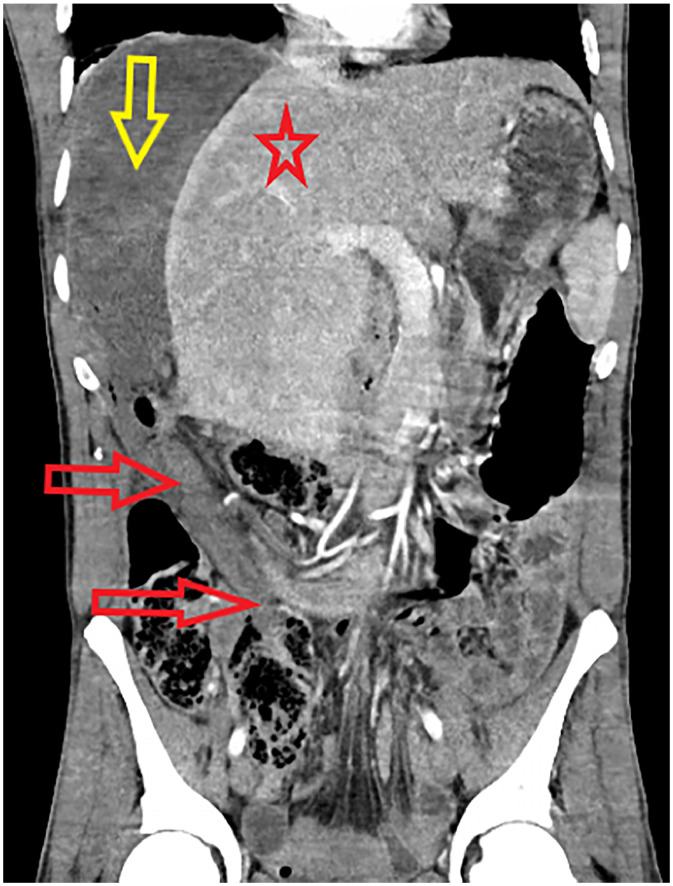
Axial contrast-enhanced CT image demonstrating a massively gas-distended colonic loop (red arrow) interposed between the liver (red star) and the right lateral abdominal wall. Multiple yellow arrows identify a curvilinear band of peripheral hepatic enhancement.
